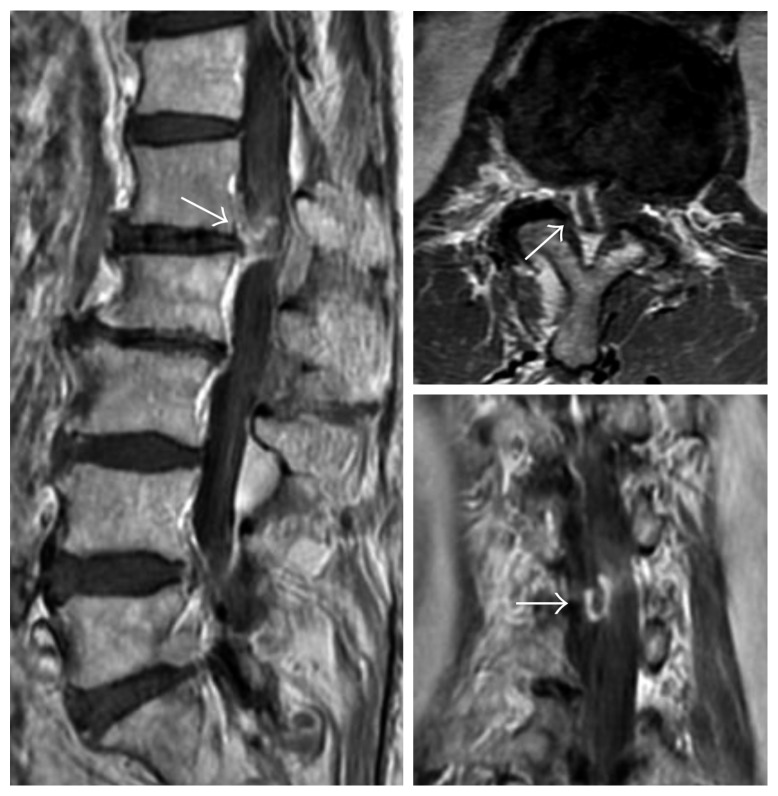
Contrast-enhanced magnetic resonance imaging: T1-weighted images show a peripheral rim enhancement of the intradural lesion (arrow).
